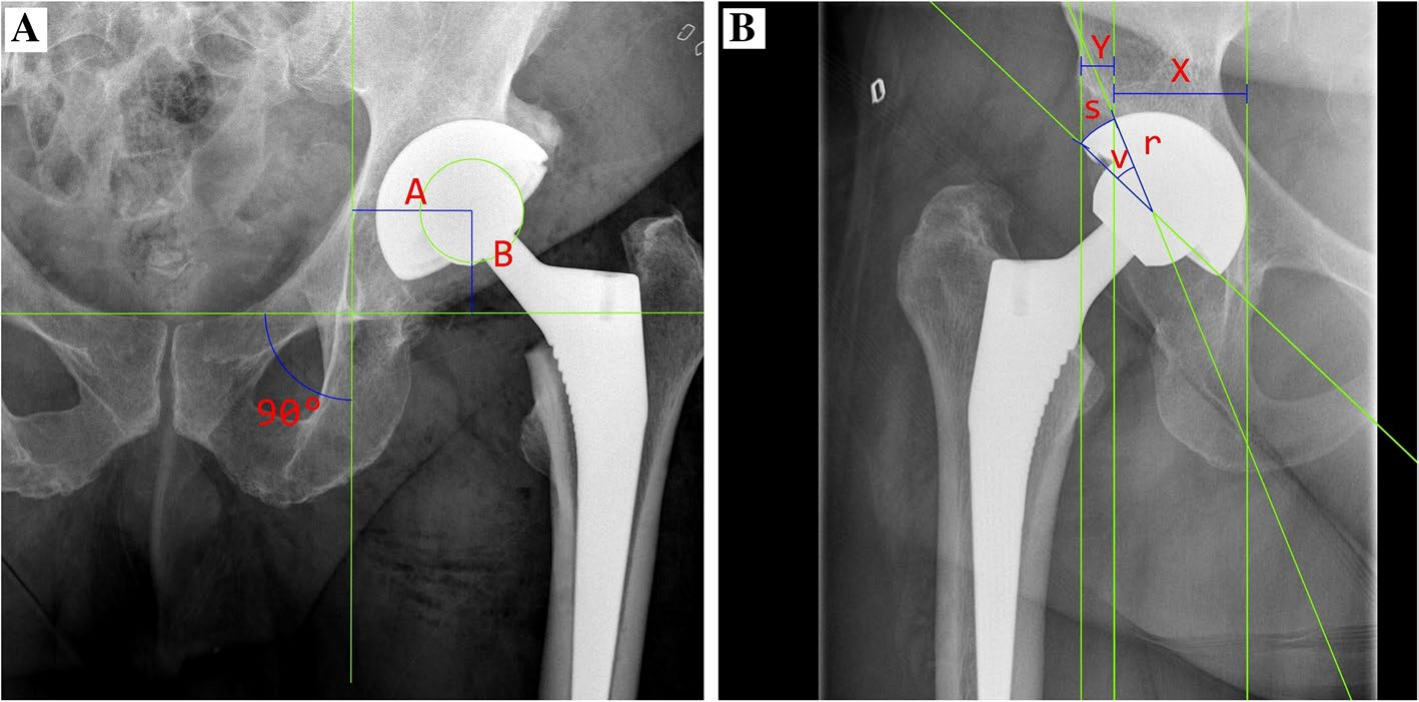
A Postoperative anteroposterior radiograph of a left Crowe type II, Hartofilakidis type B hip with measurement of location of hip centre with A the horizontal location of hip centre and B the vertical height of the hip centre. B Postoperative anteroposterior radiograph showing the assessment of projected proportion of horizontal uncovering above the acetabular component, calculated as (horizontal distance of the uncovered portion (Y) / horizontal distance between the medial and lateral edge of the implant (X)) × 100% [11]. The arc length (s) of the uncovered portion above the implant was calculated using the formula: s = (v x *r* x π) / 180, where v denotes the central angle of the uncovered portion above the implant and *r* the radius of the component [9]
